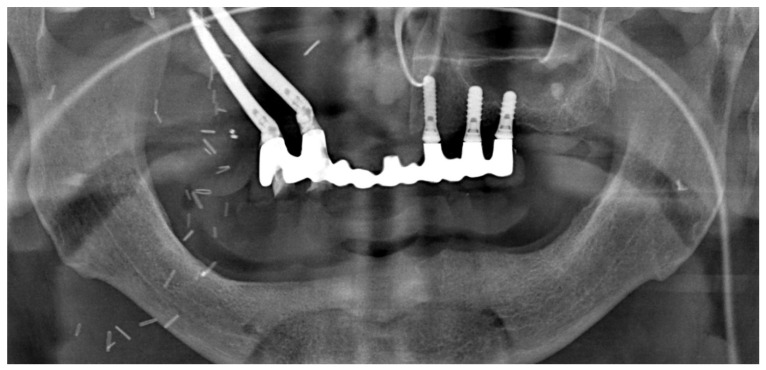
Panoramic X-ray at 7 days after surgery.
